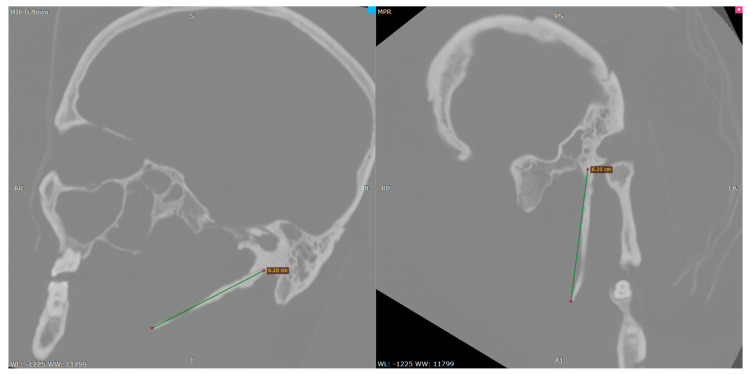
Sagittal (left) and oblique (right) multiplanar CT reconstructions demonstrating the linear measurement of the left styloid process.
